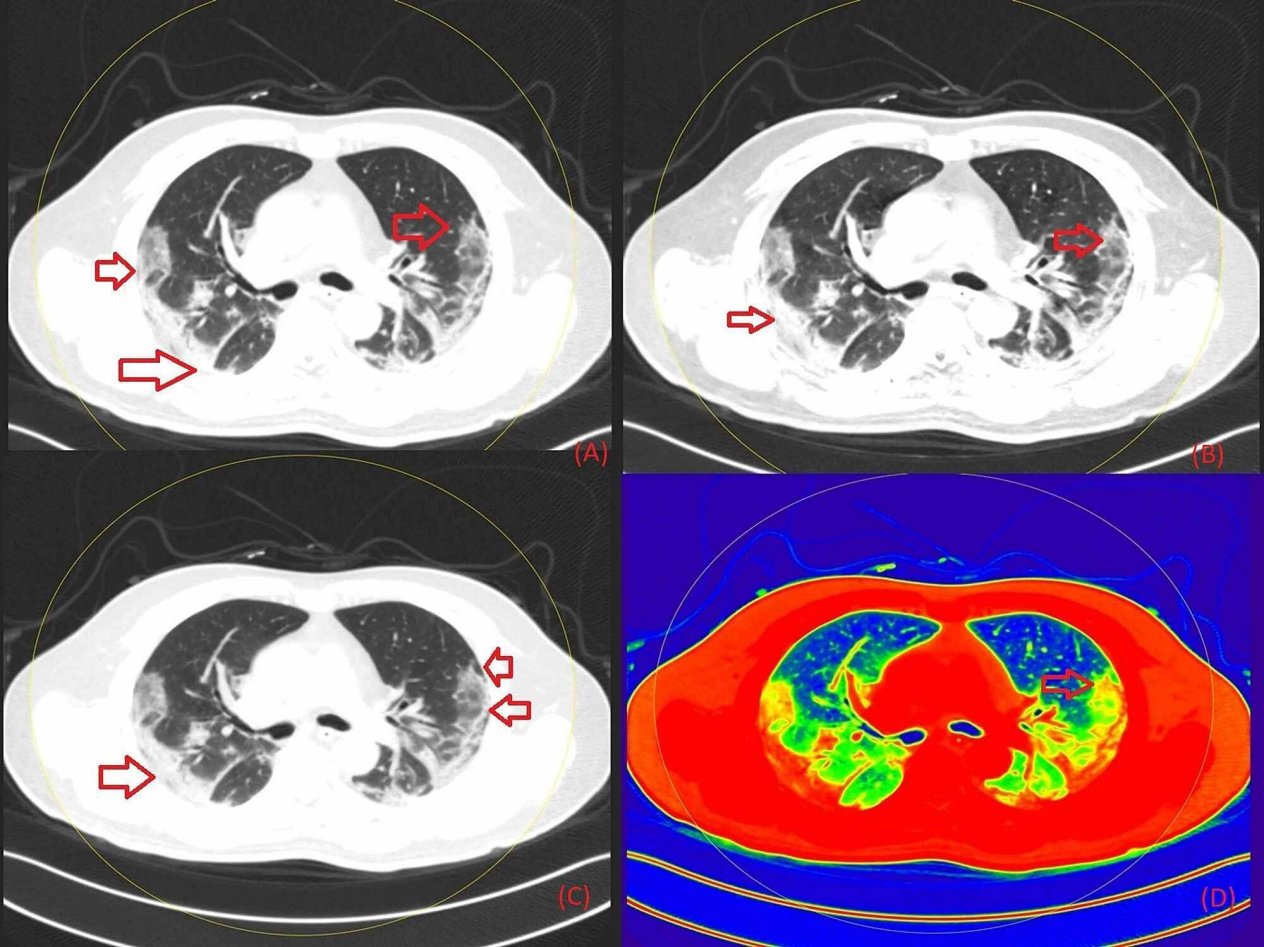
Non-contrast chest DECT axial images representing diffuse GGO (marked with red arrows) in the bilateral lung field predominantly in peripheral distribution, where Figure 2A is the section in grayscale, Figure 2B in 40 keV energy, Figure 2C in 190 keV energy, and Figure 2D as a color-coded image based on atomic number DECT: Dual-Energy Computed Tomography; GGO: Ground-Glass Opacity
